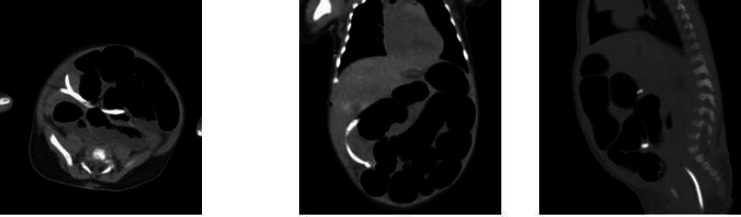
Axial, coronal, and sagittal CT views showing that the distal catheter had looped round the ilium resulting in dilated bowel and perforated rectum and migrated through anal verge.
